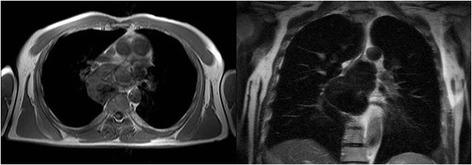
Axial T1-weighted MRI shows a well-circumscribed isointense-hypointense mass located in the posterior mediastinum, and coronal T2-weighted MRI shows a hypointense tumor located under the tracheal carina.
